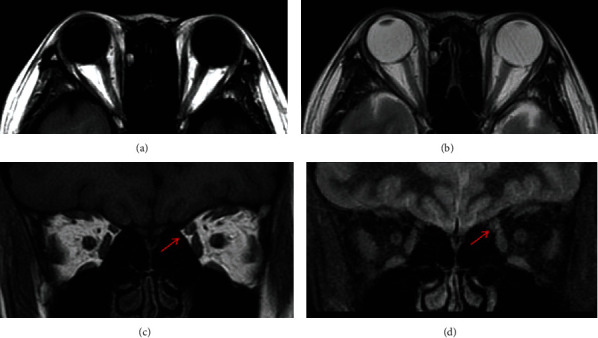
Magnetic resonance imaging (MRI) scans of the patient. The images showed a slight enlargement of the superior oblique muscle of the left eye (red arrow); however, neither enlargement nor abnormal signals were seen in the other four extraocular rectus muscles in either eye: (a) axial T1-weighted image, (b) axial T2-weighted image, (c) coronal T1-weighted image, and (d) coronal Short Tau Inversion Recovery (STIR) image.
